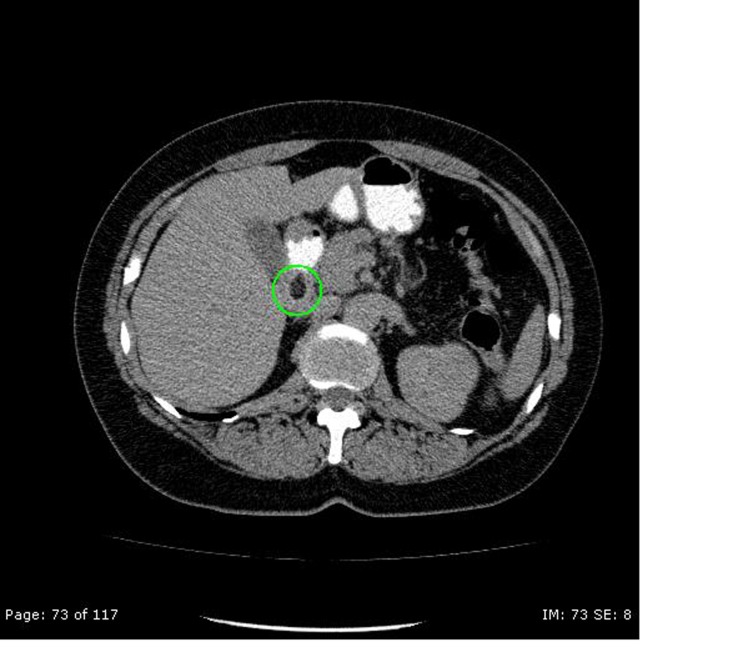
CT of the abdomen shows a T1-weighted image with an overall hypodense homogeneous low signal (-41 Hounsfield unit) lesion.
